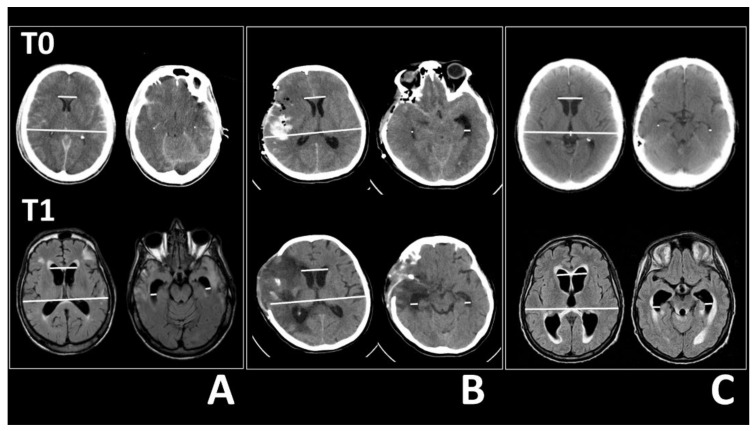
Images of CTs and MRIs acquired at admission (T0, *top row*) and at the first sign of ventricular enlargement (T1, *bottom row*) in three patients. (A) TBI patient, (B) SAH patient, (C) BT patient. In each panel, *left* images show the frontal horn distances and Evans’ index measurements (*white lines*), and *right* images show the temporal horn width measurements (*white lines*). (A) Compared to the CT images (T0), the MRI scans after 8 weeks (T1) shows that the frontal horns enlarged from 29.1 to 39.2 mm and the Evans’ index increased from 0.22 to 0.3 (*left*). The right temporal horn enlarged from 1.4 to 7.7 mm and the left temporal horn enlarged from 0.9 to 7.3 mm (*right*). (B) T0 image is scanned after clipping a middle right cerebral artery aneurysm and performing a decompressive craniectomy for SAH. A CT image acquired 19 days after surgery (T1) shows that the frontal horn distance enlarged from 30.1 to 37.7 mm and Evans’ index increased from 0.22 to 0.28 (*left*). The right temporal horn width enlarged from 1.9 to 9.1 mm and the left temporal horn width enlarged from 7.7 to 8.2 mm (*right*). (C) T0 CT scan shows a patient with fourth ventricle lymphoma. After 3 weeks (T1), the MRI shows an enlarged frontal horn distance (from 33.6 to 43.5 mm) and an increase in Evans’ index, from 0.25 to 0.32 (*left*). The right temporal horn width enlarged from 1.3 to 10.9 mm and the left temporal horn width enlarged from 1.7 to 11.4 mm (*right*).
